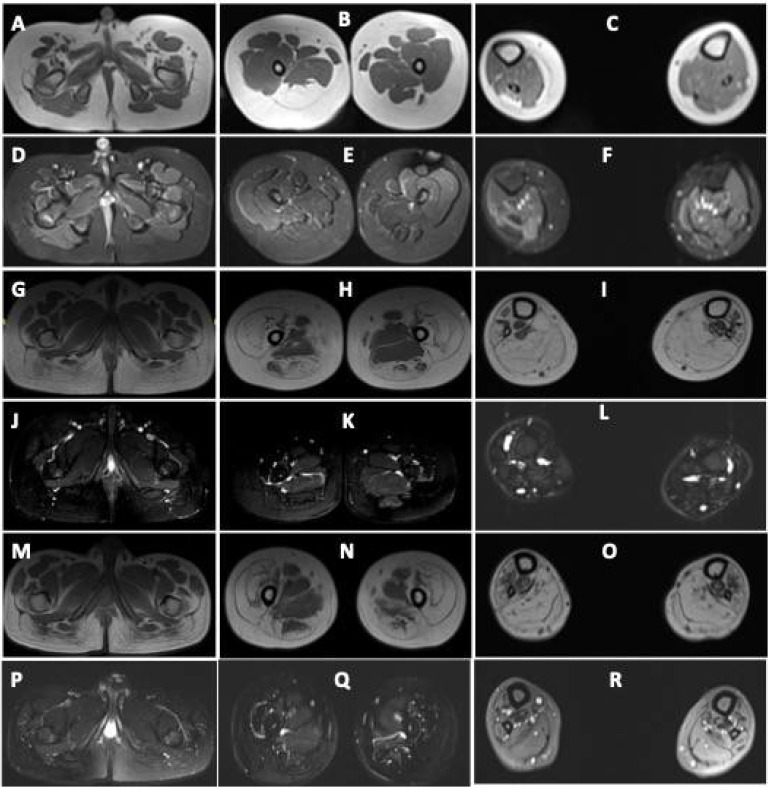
Muscle MRI images of patients with DA5D: (A–F) muscle MRI images of patient 1: axial T1-weighted sections at the level of the (A) pelvis, (B) midthigh, and (C) midleg reveal volume loss with fatty replacement, most pronounced in bilateral tensor fascia lata (Mercuri Grade 3), right semimembranosus (Grade 3), semitendinosus (Grade 3), bilateral biceps femoris (Grade 2b). Grade 2a fatty replacement is seen in the lateral compartment of the right leg and gastrocnemius. Axial fat-saturated T2-weighted sections at the level of the (D) pelvis, (E) midthigh, and (F) midleg reveal no edema; (G–L) MRI images of patient 2 and (M–R) of patient 3: axial T1-weighted sections at the level of the (G,M) pelvis, (H,N) midthigh, and (I,O) midleg reveal volume loss with fatty replacement, most pronounced in bilateral gluteus maximus (Mercuri Grade 3), vasti (Grade3), rectus femoris (Grade 2a), semimembranosus (Grade 2b), semitendinosus (Grade 2b), biceps femoris (Grade 2b). Grade 2b fatty replacement is seen in the anterior, lateral, and deep posterior compartments of the legs. The superficial posterior compartment of both legs reveals Grade 4 atrophy with fatty replacement. Axial fat-saturated T2-weighted sections at the level of the (J,P) pelvis, (K,Q) midthigh, and (L,R) midleg reveal no fluid signal.
